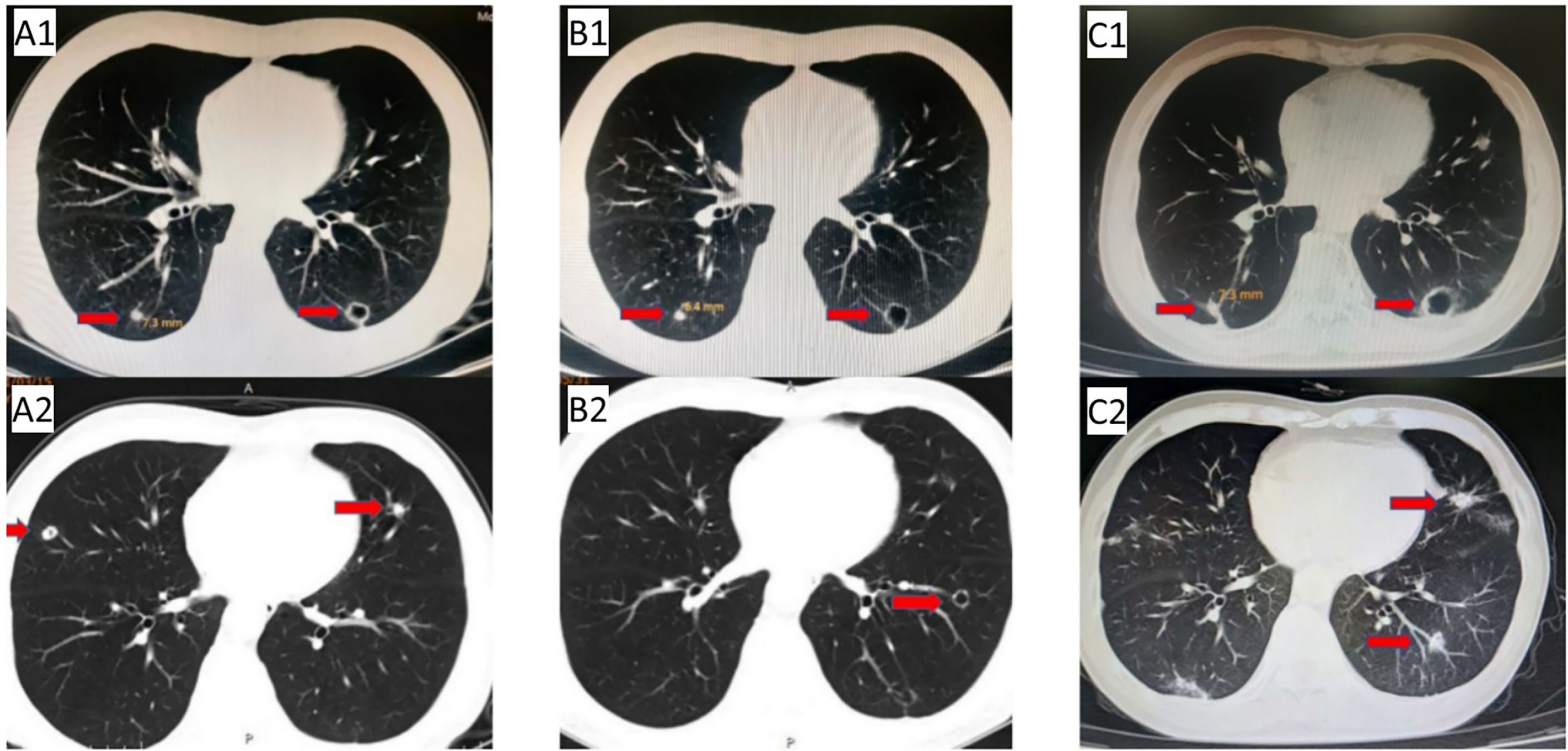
Anlotinib + TAS-102 treatment:(A1/A2) CT after 2 cycles (2021.03.15): the pulmonary nodules were larger than before (the right pulmonary nodules was 7.3 mm), and some cavities were formed; (B1/B2) reexamination CT (2021.05.31) showed that the right pulmonary nodules were smaller than before (6.4 mm); (C1/C2) last reexamination CT (2022.08.23): the right pulmonary nodules was 7.3 mm; multiple nodules in the left lung, and some cavities were formed.
